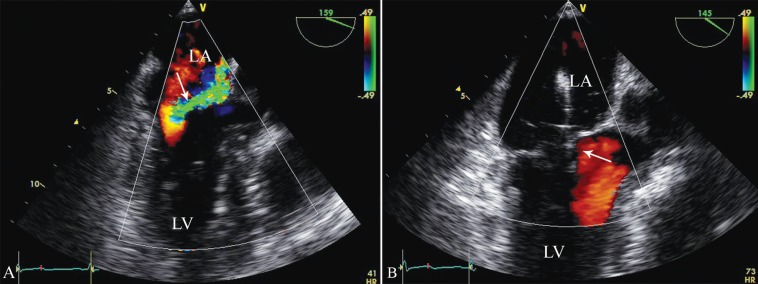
Echocardiographic view, showing severe mitral regurgitation in color Doppler (arrow) (A), which is reduced to mild mitral regurgitation (arrow) after device implantation (B)
